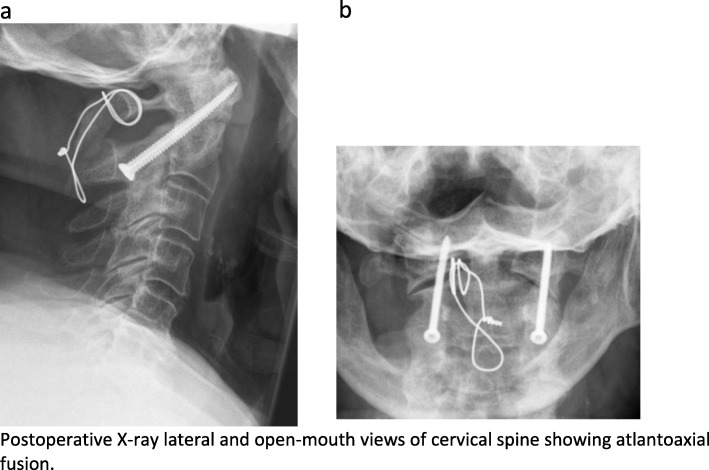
a, b Postoperative X-ray lateral and open-mouth views of the cervical spine showing atlantoaxial fusion
